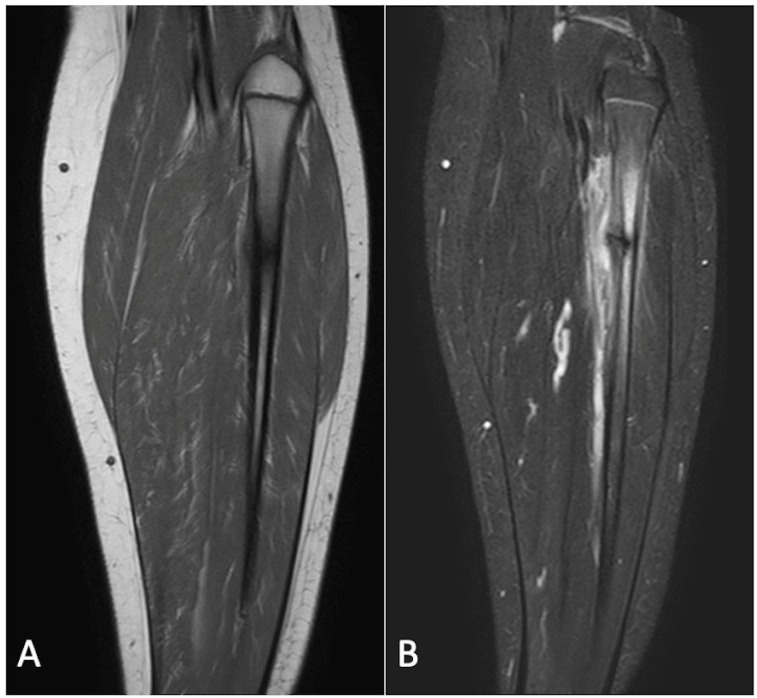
T1 (A) and STIR (B) coronal MRI images demonstrating stress fracture of the proximal fibula with intramedullary, periosteal and extraosseous oedema, along with a low signal fracture line.
